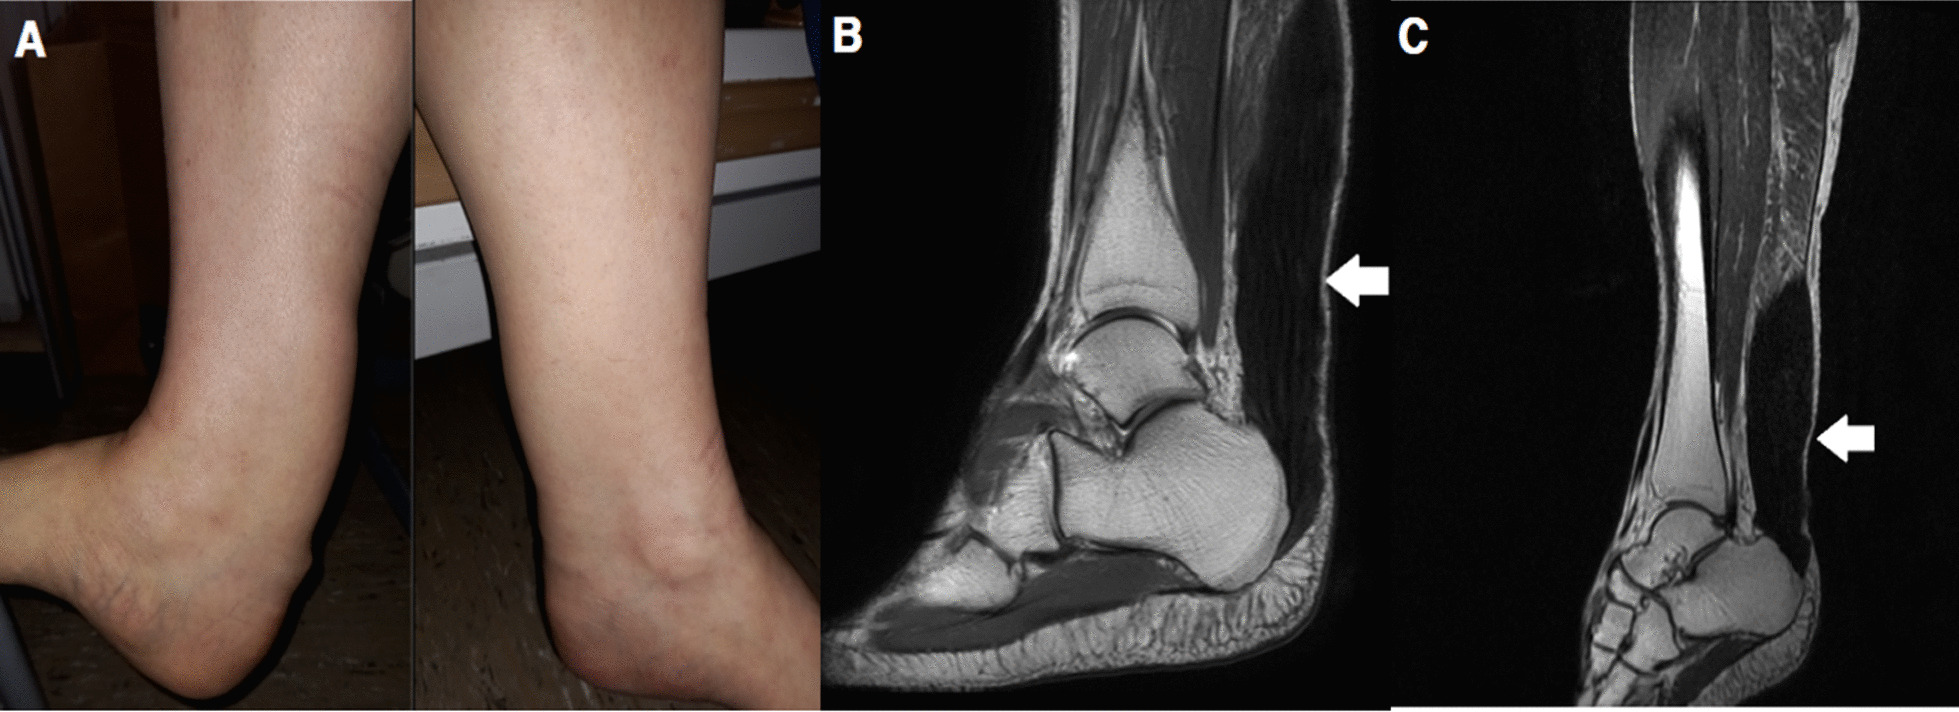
A Bilateral masses on the patient’s Achilles tendons. B, C Ankle MRI (T2-weighted image) revealed tendinous xanthomas on the patient’s both Achilles heels (white arrows), (B): left, (C): right
